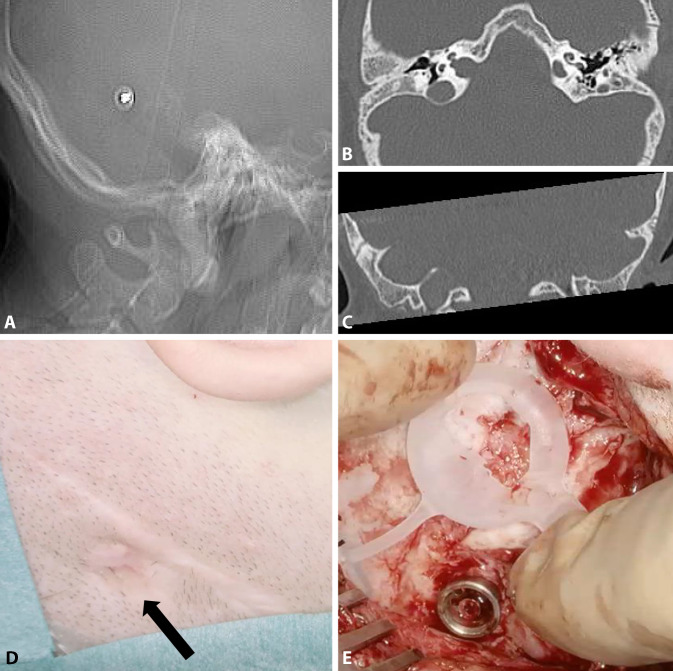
Radiological planning and surgical field for the simultaneous explantation of a fixture from a percutaneous bone conduction implant and implantation of a BCI 602 (right ear). A The computed tomography (CT) scout view shows the fixture. Axial CT (B) and coronal (C) CT show mastoid hypoplasia, the lateralized sigmoid sinus, and minimal cortical bone thickness (<5 mm) at the bone conduction-floating mass transducer (BC-FMT) position. The abutment is completely overgrown with skin (*black arrow*) (D) and is removed before creating the implant bed (E). (A–C: CT images reproduced with permission from Prof. Dr. med. M.A. Weber, Institute for Diagnostic and Interventional Radiology, Pediatric and Neuro-Radiology, University Medicine Rostock)
